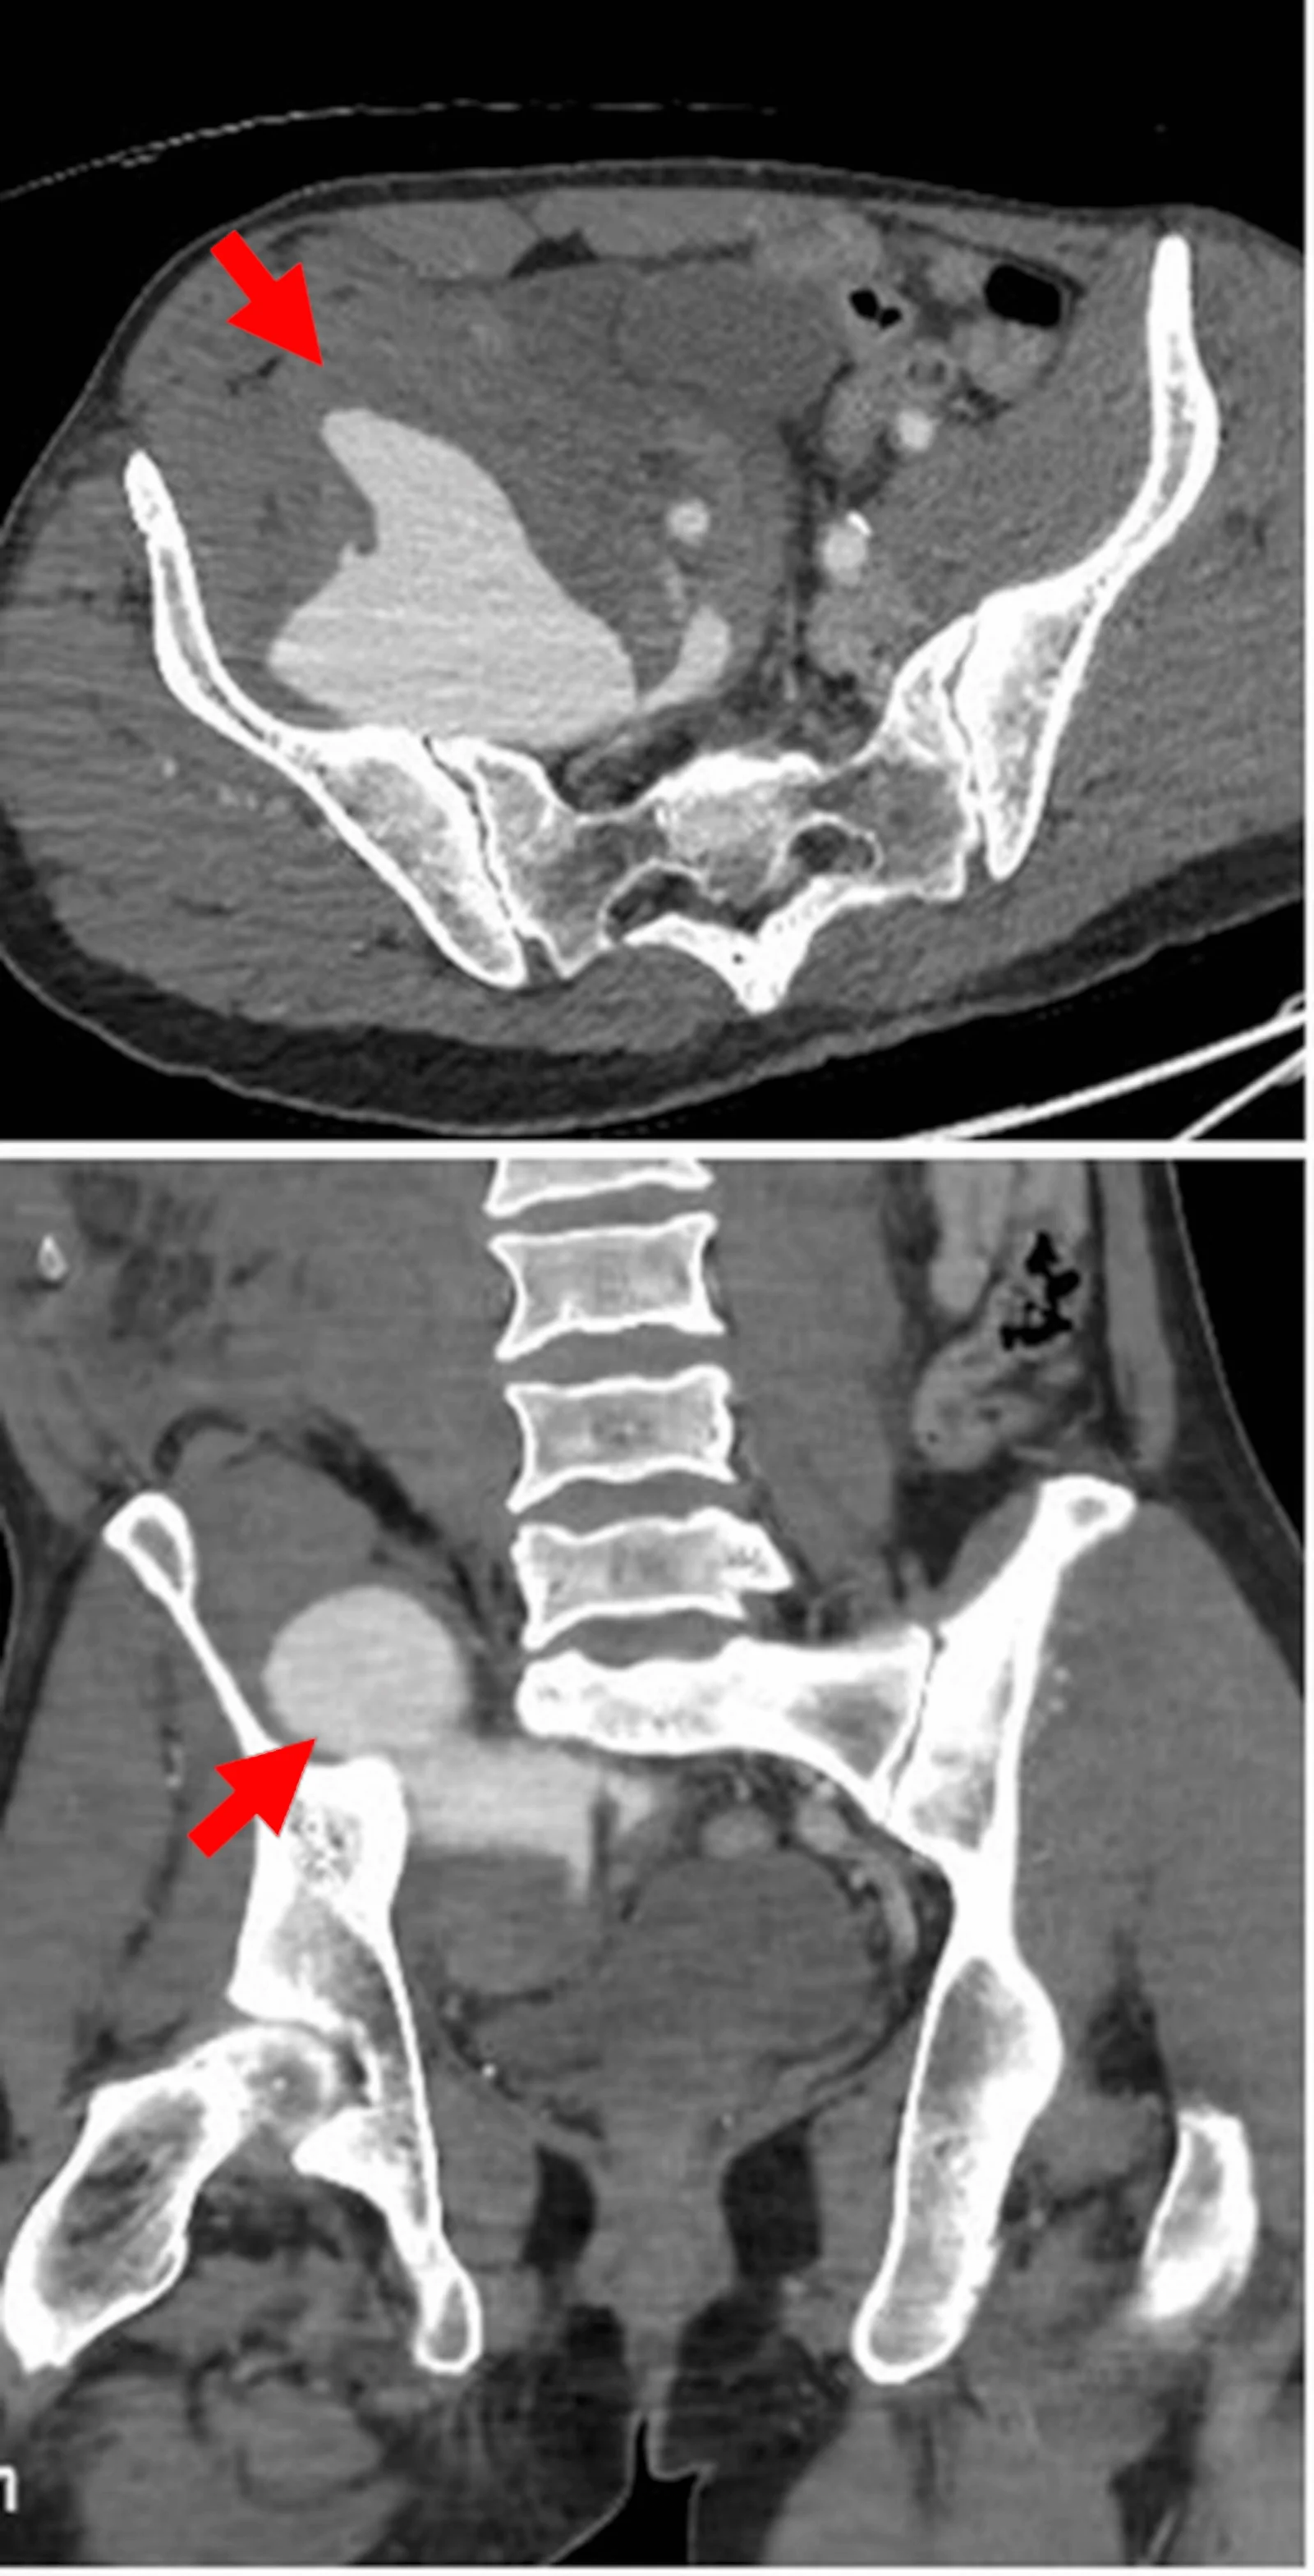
Ruptured right internal iliac artery aneurysm CT: computed tomography Contrast-enhanced CT (axial and coronal views) showing an 88 mm ruptured aneurysm arising from the right internal iliac artery, with an extensive right retroperitoneal hematoma indicating active rupture. The red arrow indicates the ruptured internal iliac artery aneurysm
